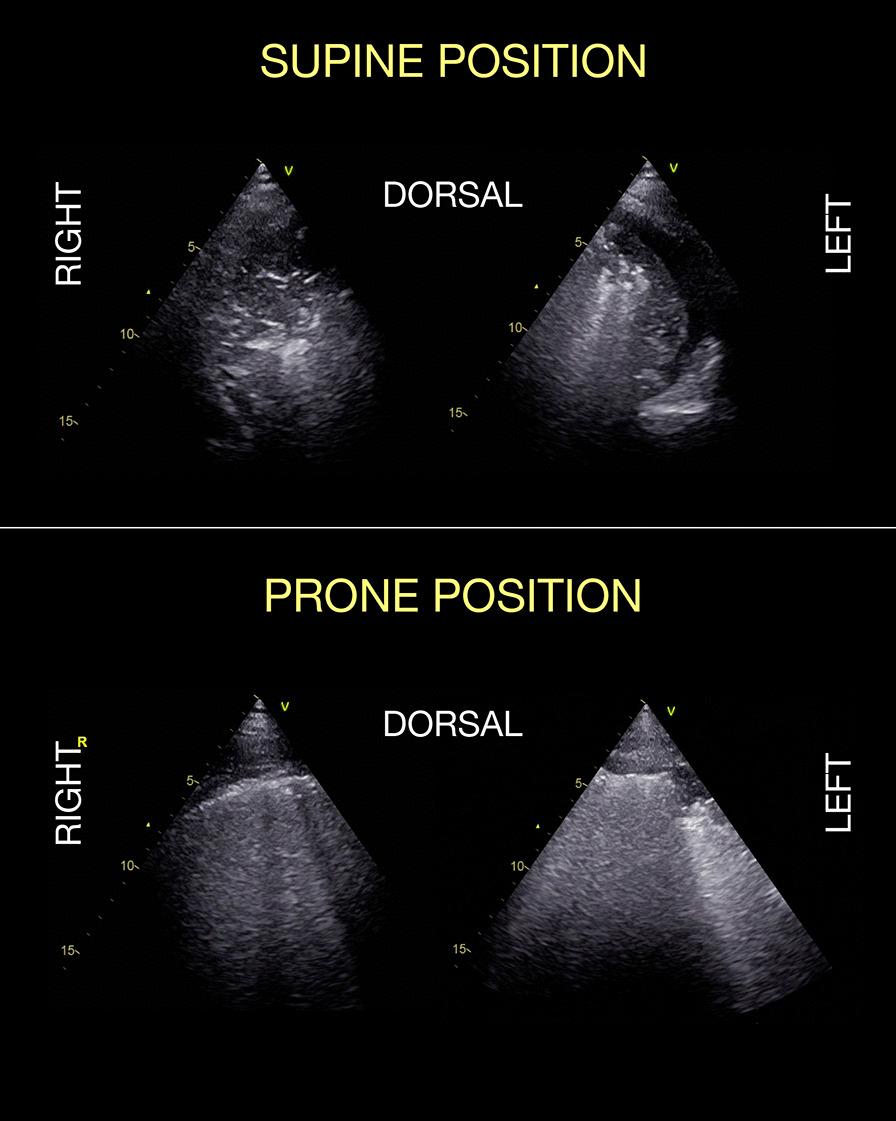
Lung ultrasound to monitor adequacy of re-aeration of dorsal areas upon pronation and recruitment maneuvers in a COVID-19 patient. Same patient of Fig. 2B, before (upper panels) and after (lower panels) pronation and a series of stepwise recruitment maneuvers up to PEEP 26 cmH_2_O, and final PEEP setting at 16 cmH_2_0
